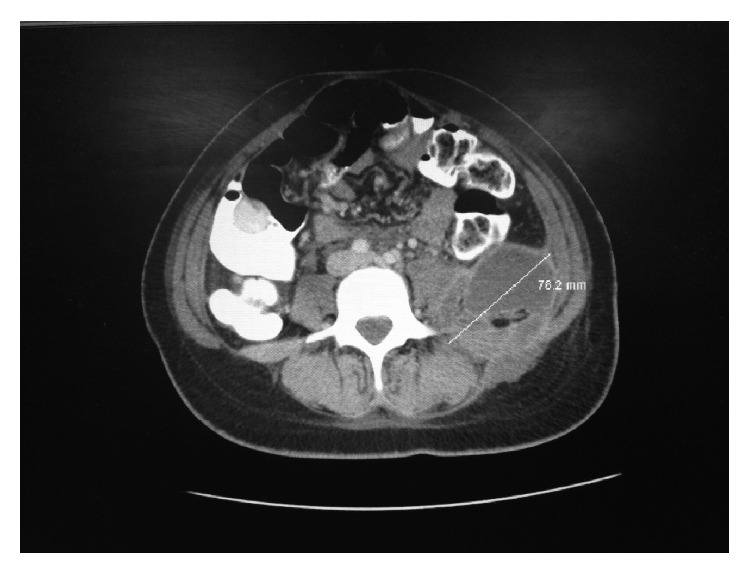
CT of abdomen/pelvic revealing a 7.6 × 5 cm left colonic abscess.
